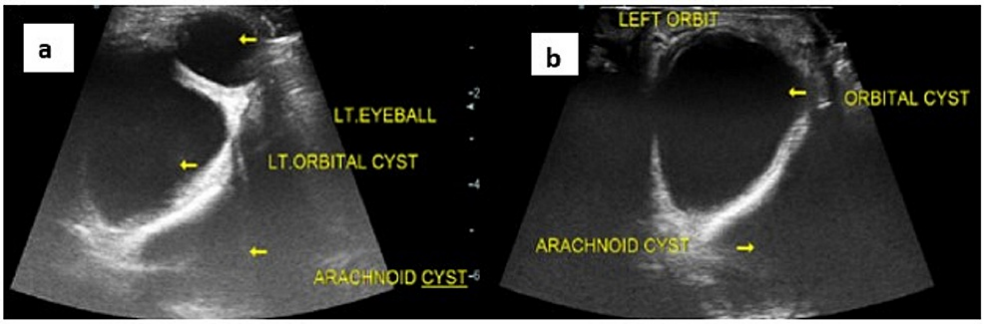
(a) Ultrasound examination of the left orbit showing well-defined anechoic cystic lesion without solid component, septations, or calcification and intracranial cyst behind the left sphenoid wing without solid component, septations, or calcification. (b) Ultrasound examination cranially to the previous image showing intraorbital cyst and intracranial cyst in the left middle cranial fossa.
